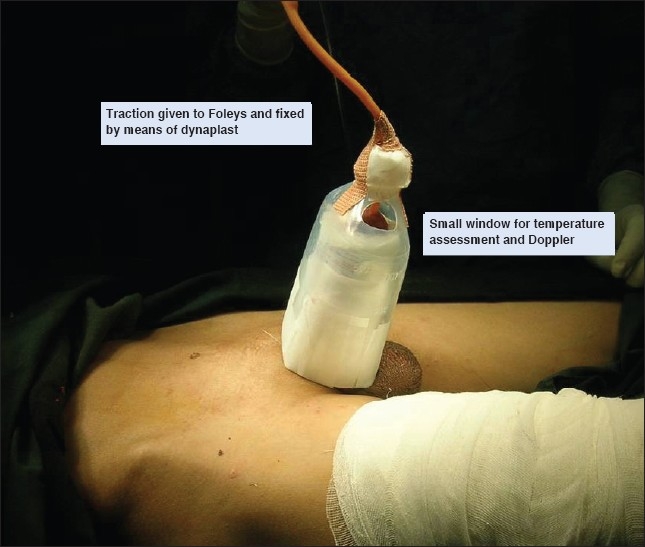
Splint showing window for palpation and/or doppler
